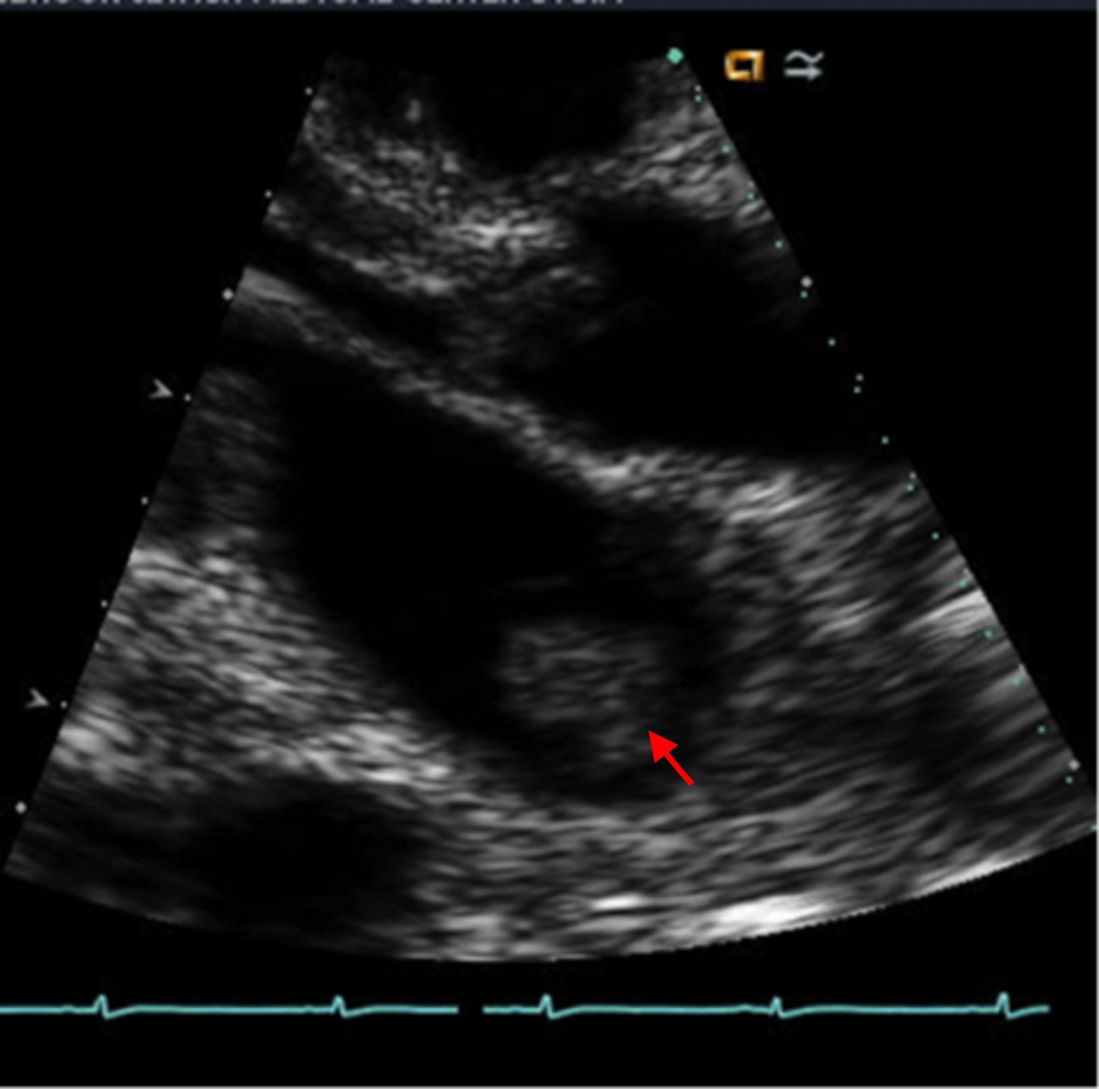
Transthoracic echocardiogram showing the left atrium mass
